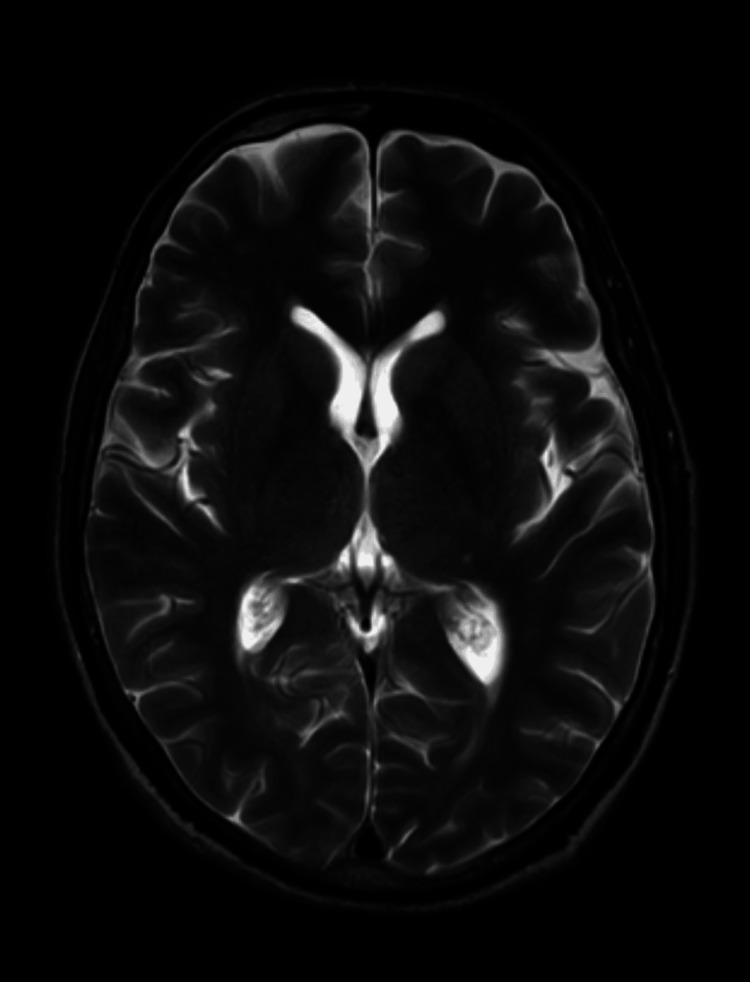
MRI - T2-weighted axial section of the brain suggesting normal imaging study MRI: magnetic resonance imaging
